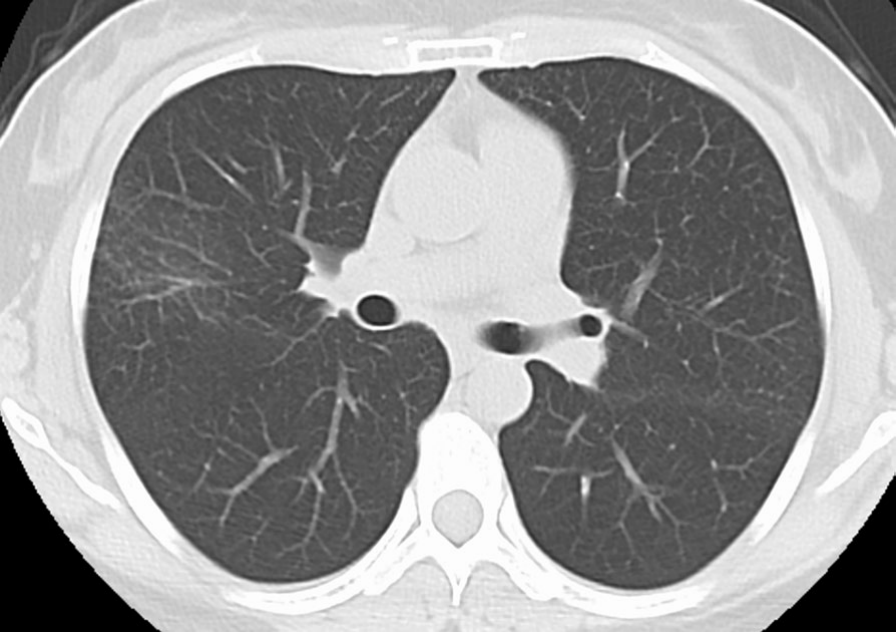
The nodular lung lesions disappeared after discontinuation of IFX-BS in CT scan
